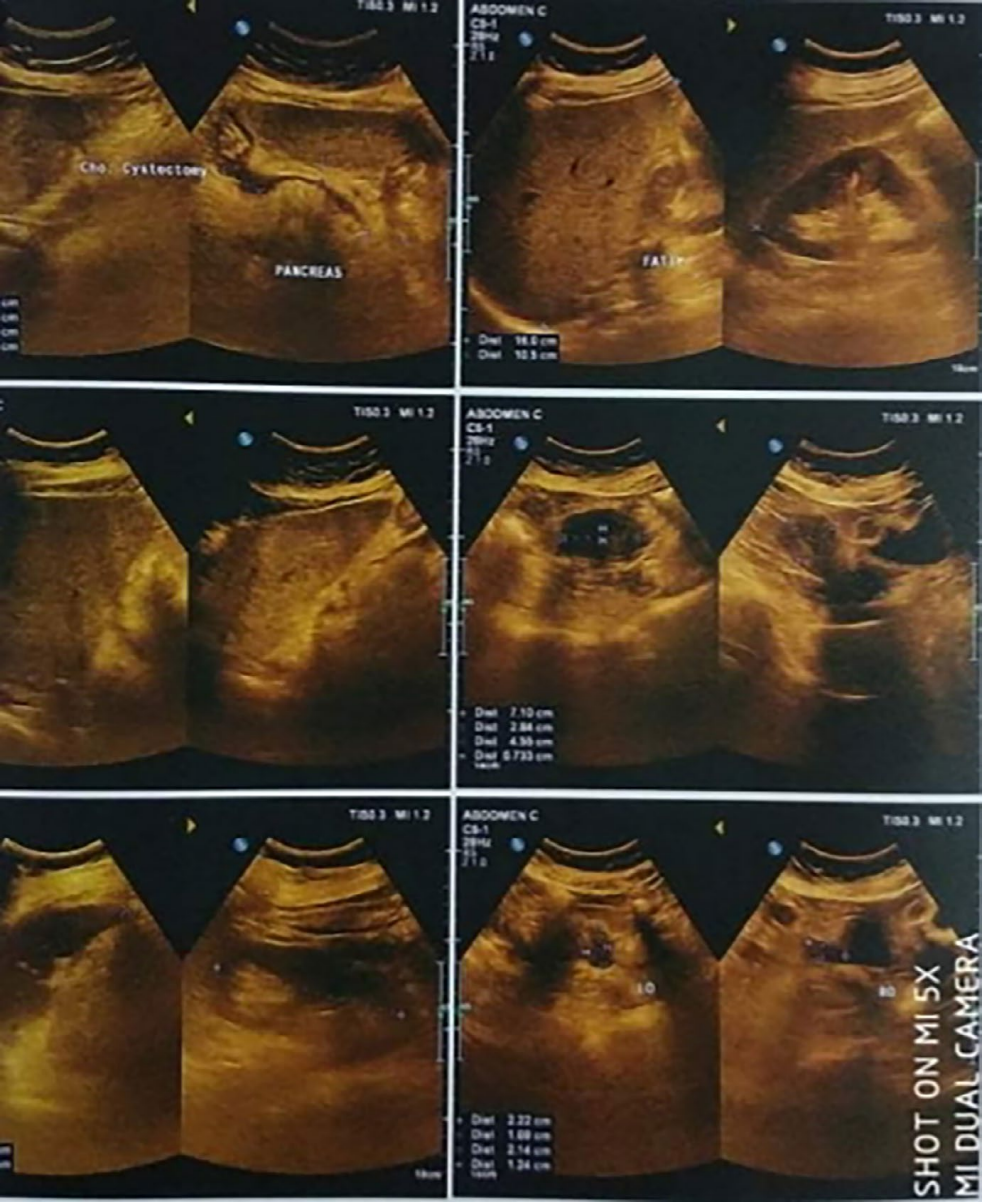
Follow‐up Ultrasonography of Abdomen
